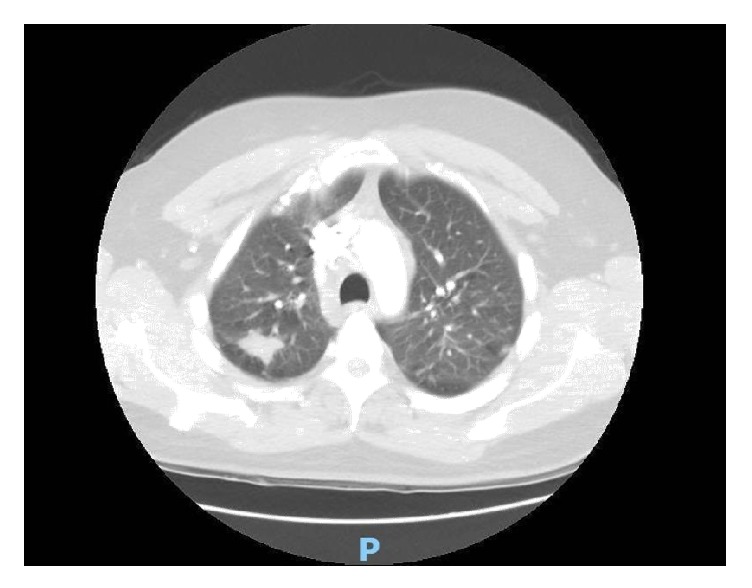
CT lung depicting right upper lobe lung nodule (Case 1).
